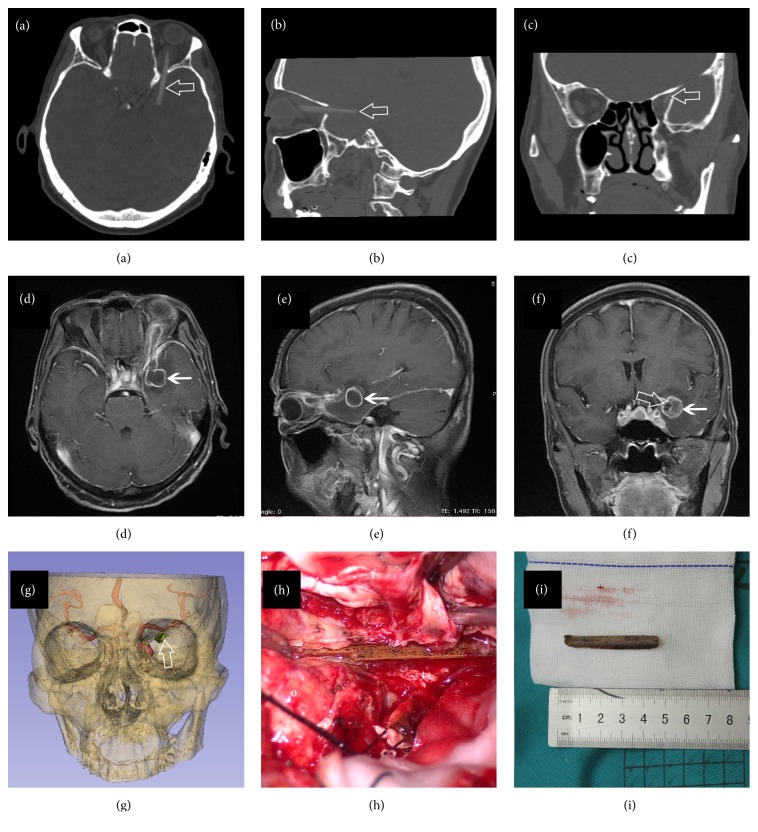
Head CT scan demonstrated a bamboo stick (hollow arrow, ⇦) penetrating into the temporal lobe via superior orbital fissure. The bamboo stick presented as high density on the CT scan (a, b, c). Contrast enhanced MRI revealed an abscess (simple arrow, *←*) around the bamboo stick in temporal lobe (d, e, f). 3D reconstruction of the skull, cerebral artery, and bamboo stick (hollow arrow, ⇦) was performed by 3D Slicer software to visualize the relationship among these structures (g). Intraoperative photography (h) displayed the bamboo stick in original place (o, orbital side; f, frontal side; t, temporal side). Photography showed the removed bamboo stick (i). 3D, three-dimensional; CT, computed tomography; MRI, magnetic resonance imaging.
